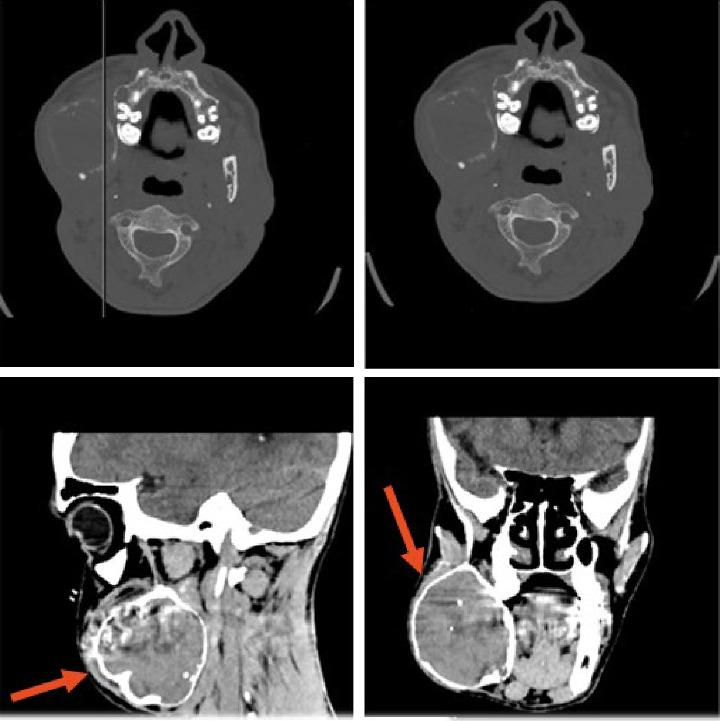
CT of the mandible. It shows an expansive lytic lesion in the right mandibular bone body.
